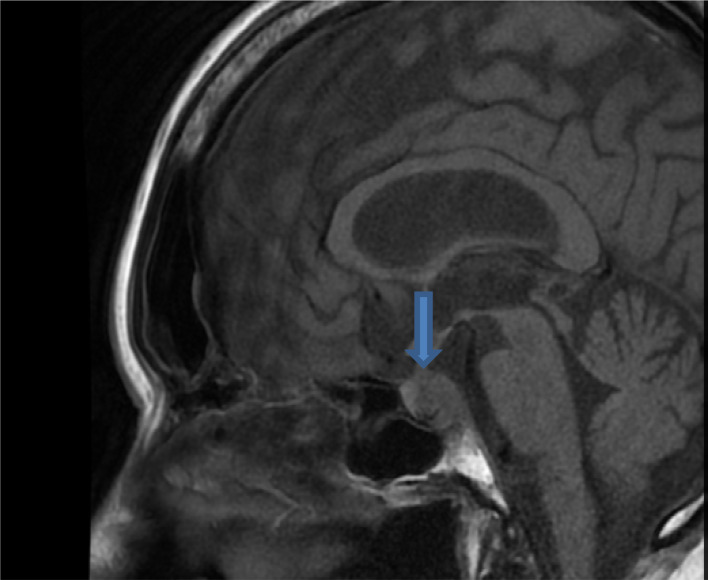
Case 4. Magnetic resonance image of the pituitary gland showing a pituitary stalk lesion.
